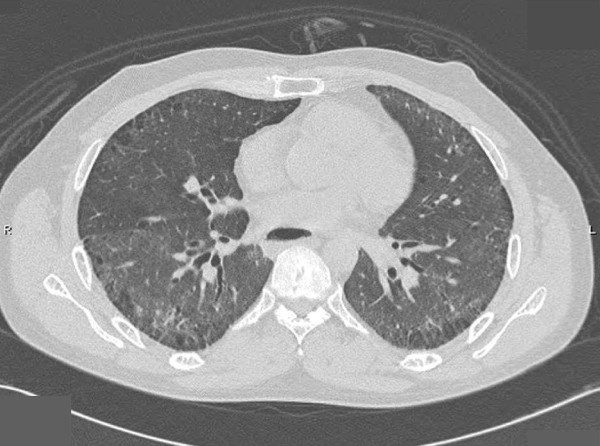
HRCT chest scan revealed minimal thickening of the interlobular septa with thickening of the pleura in both lungs and mild bronchiectasis in both lower lungs.
